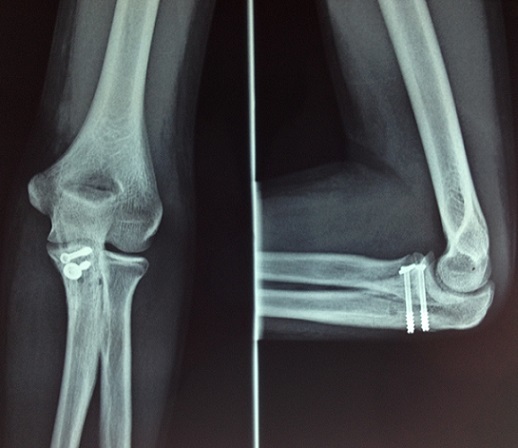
(a, b) radiographie du coude gauche de face et de profil: consolidation du processus coronoïde au recul de deux mois
